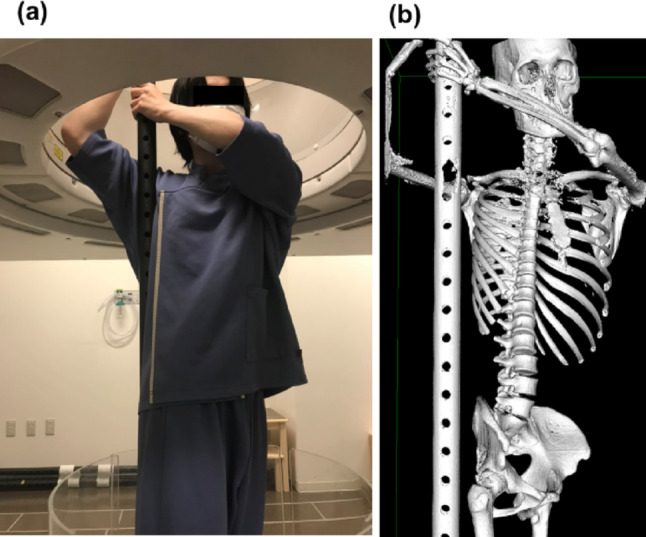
Methodology for acquiring maximal trunk rotation scans using upright CT. a Photograph of a healthy volunteer positioned inside the upright CT gantry, standing with both feet aligned on designated foot markers, performing maximal right trunk rotation while gripping a central stabilizing bar. b Corresponding three-dimensional (3D) volume-rendered image reconstructed from the scan obtained in this posture
